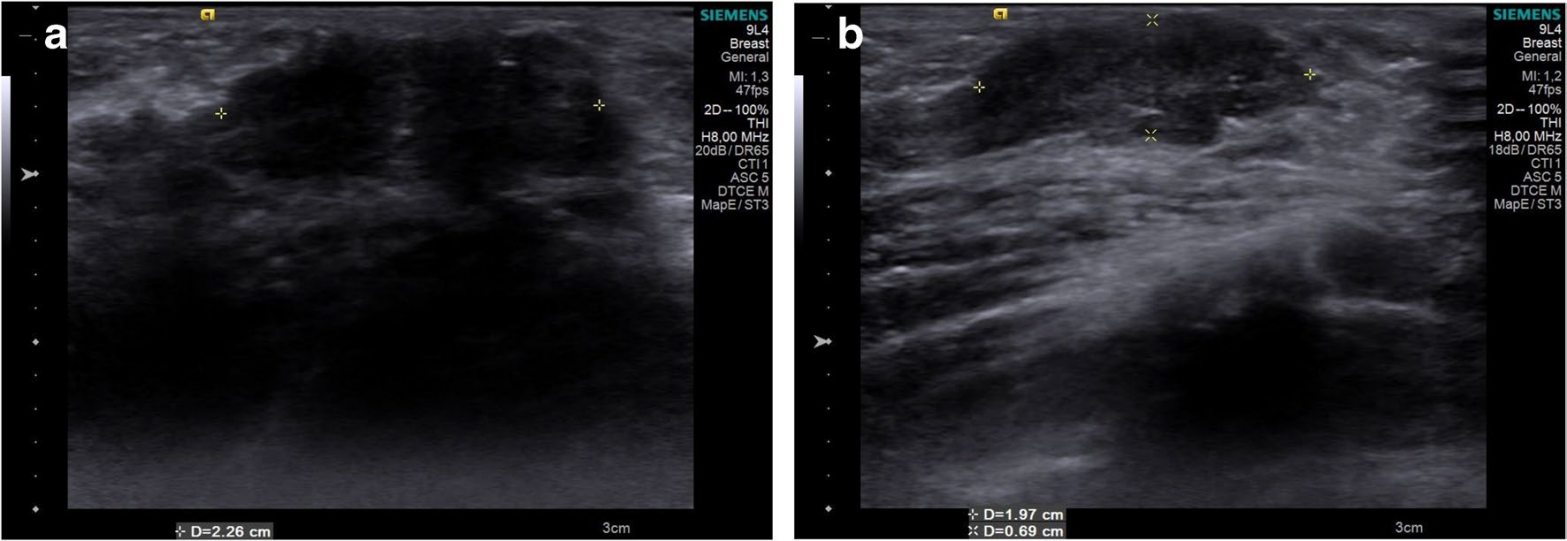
Grayscale ultrasound volume measurements of a stage III breast bud in a 4-year-old girl who was diagnosed with premature thelarche. a Longitudinal plane image shows the superoinferior measurement (*calipers*). b Axial plane image shows the mediolateral (*calipers* +) and anteroposterior (*calipers* x) measurements. Breast volume was calculated to be 1.6 cc
